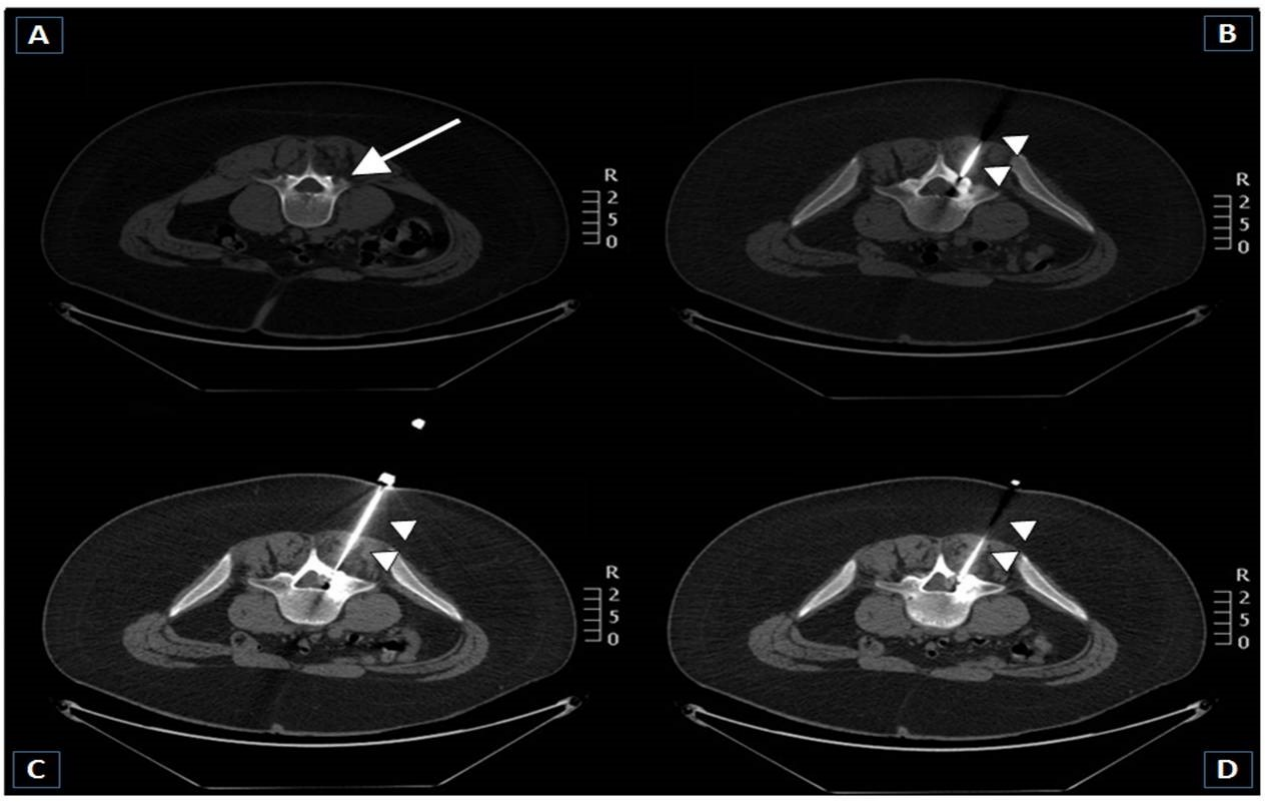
15-year-old male patient affected by an osteoid osteoma in the left lamina of the posterior arch of L4. A–Axial CT image shows an osteoidosteoma in the external aspect of the posterior arch of L4. The diameter of nidus is 7 mm. B, C, D–Axial CT-guided RFA scans show the correct position of the tip of the RF electrode within the center of the nidus (technical success). (c) Ablation was successfully performed. The risk was the possibility of affection of the nerves belonging to the caudaequina and the left L5 nerve root by thermal injury. However, this did not happen as the size of the active electrodetip was exactly equal to the diameter of the nidus and the whole thermal energy was contained within the nidus. The patient was discharged symptom-free two days after the procedure.
